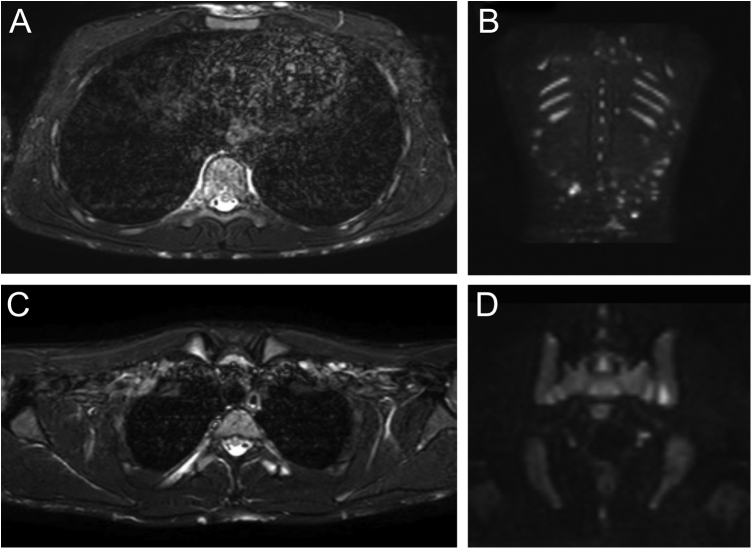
Patient 1: Axial Short Tau Inversion Recovery (STIR) MRI images (left) of the chest revealing nodular hyperintensity signals in the cutaneous-subcutaneous region affecting the anterior chest wall as well as the back (A); hyperintensity signal of the posterior arch of the fourth rib on the right due to marrow edema but no edema of the surrounding soft tissues (B). Coronal Diffusion-Weighted Whole-Body Imaging with Background Body Signal Suppression (DWIBS) images (right) demonstrating increased signal at the level of multiple subcutaneous alterations of the patient's back (C); focal hyperintensity signal at the left sacroiliac joint (D).
